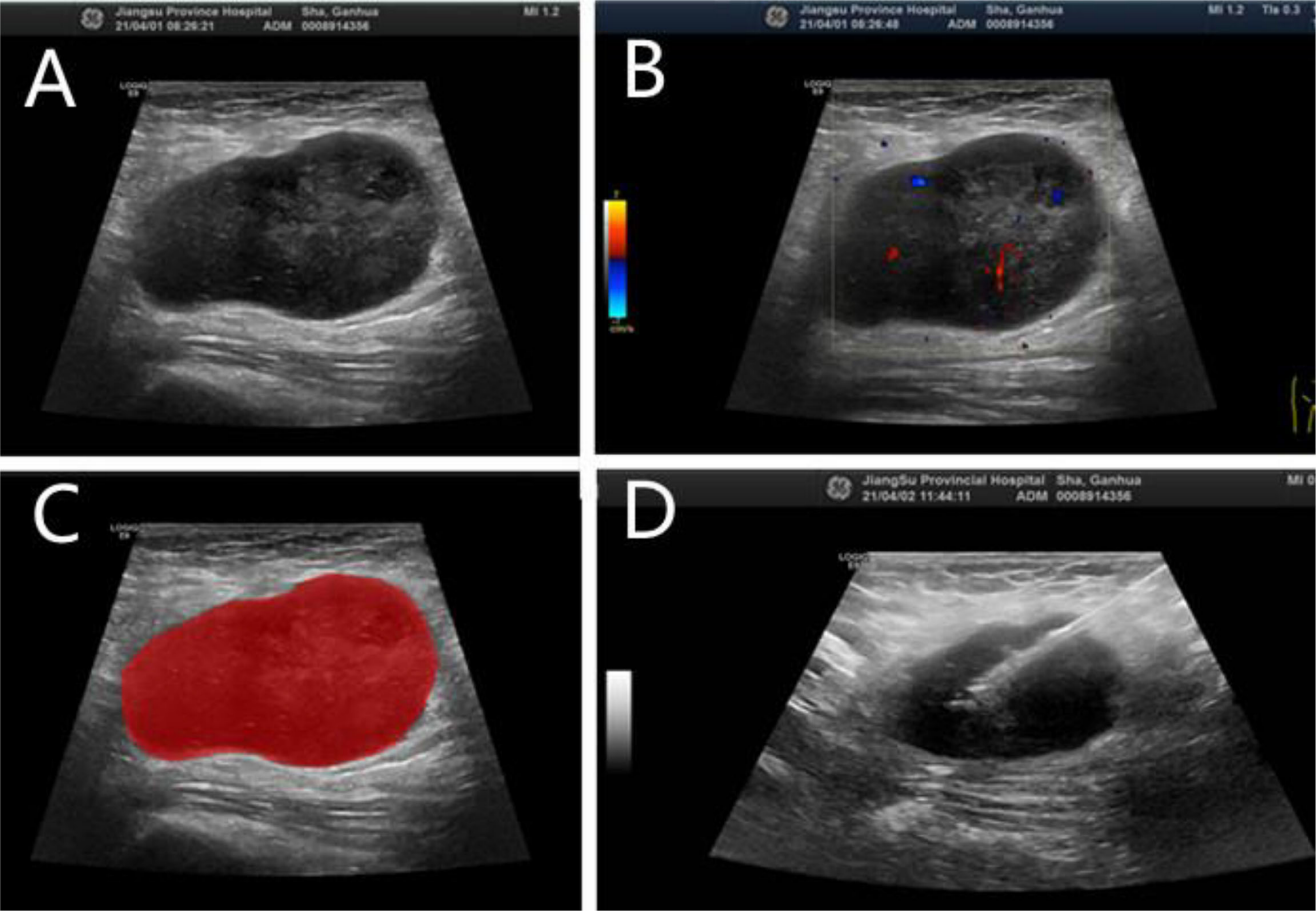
N-DLBCL in a 58-year-old man. (A) Gray-scale ultrasonic image. (B) CDFI image. (C) Radiomic ROI segmentation segmentation of the mass. (D) Ultrasound-guided core needle biopsy.
